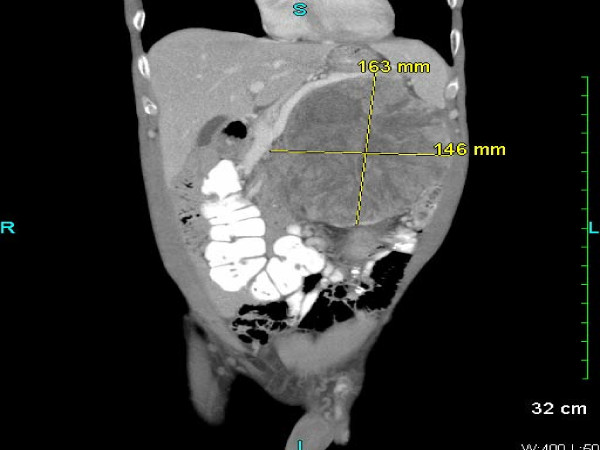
Sagittal view of abdominal computed tomography (CT) examination of the patient. This abdominal CT scan shows a large retroperitoneal mass displacing the left kidney inferiorly and the spleen superiorly.
